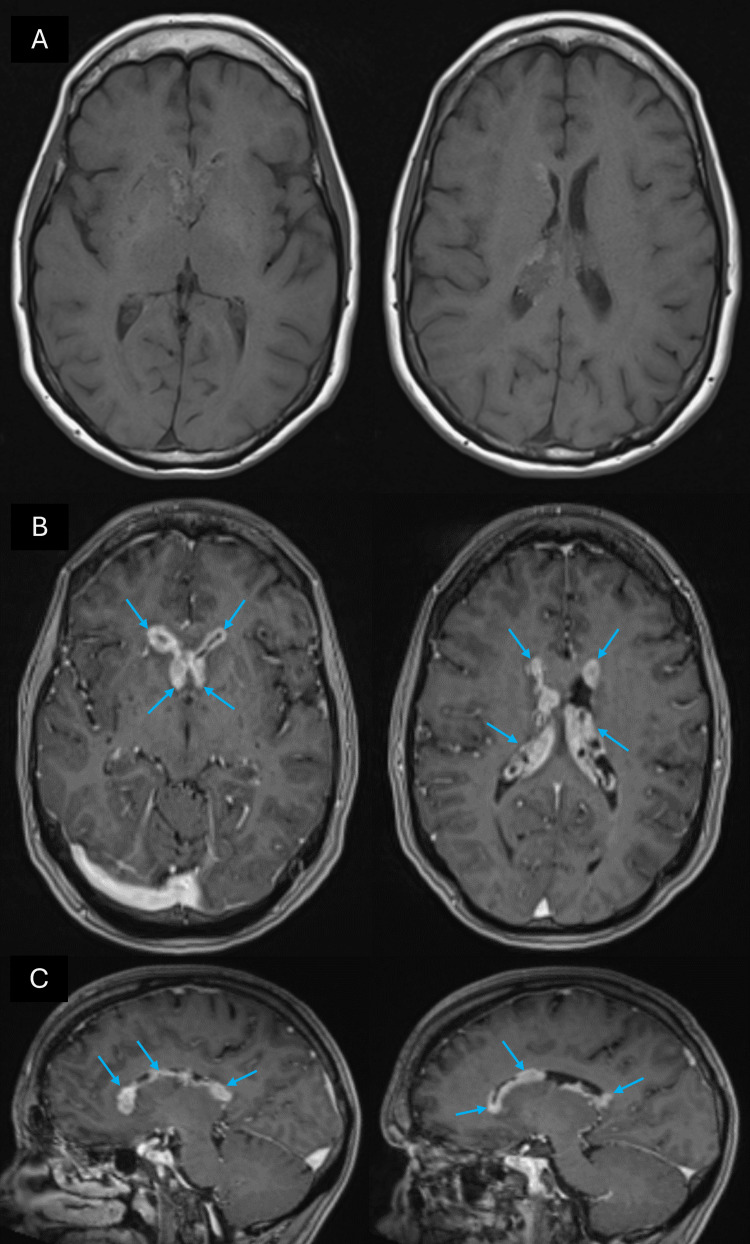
MRI at initial presentation (A) Axial T1 MRI without contrast and (B) Axial, (C) Sagittal T1 MRI with contrast showing diffuse ependymal enhancement of the lateral ventricles bilaterally (blue arrows)
